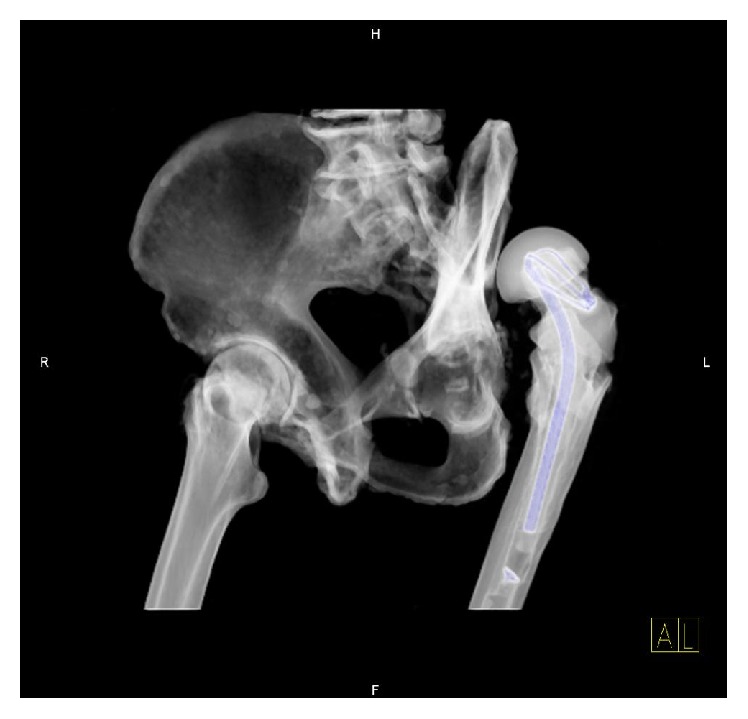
3D CT reconstruction showing a dislocated left-sided THR secondary to a posterior acetabular wall deficiency.
